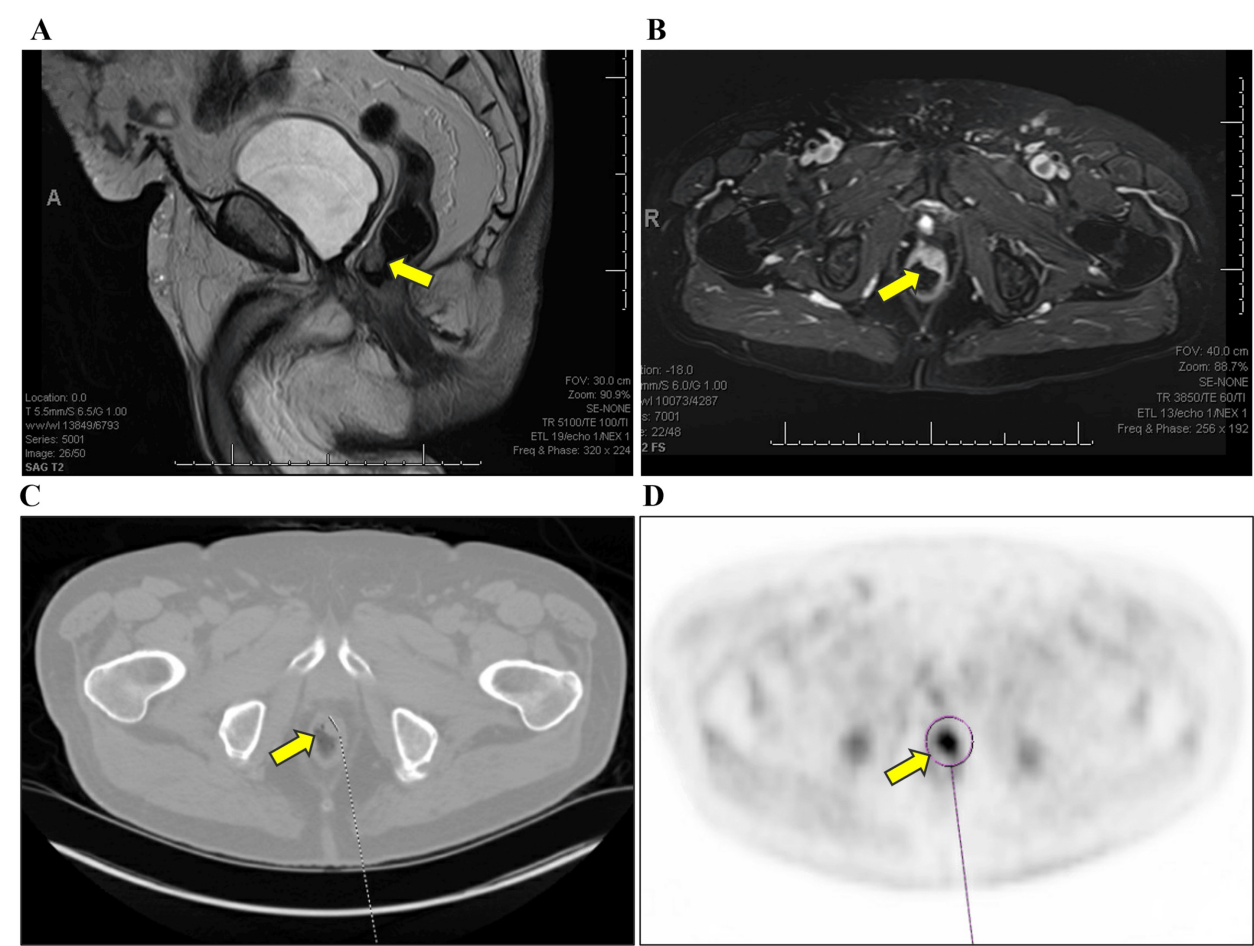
Imaging studies of the rectal polypoid lesion. A and B: MRI showing a solitary polypoid lesion; C and D: PET scan showing a hypermetabolic soft tissue mass in the anterior left rectal wall.
